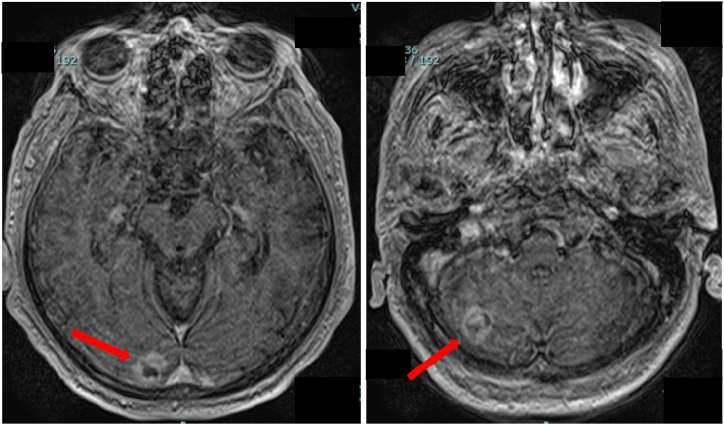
Contrast-enhanced MRI of the head. Multiple brain metastases are observed in the cerebral and cerebellar hemispheres (arrows) MRI, magnetic resonance imaging.
